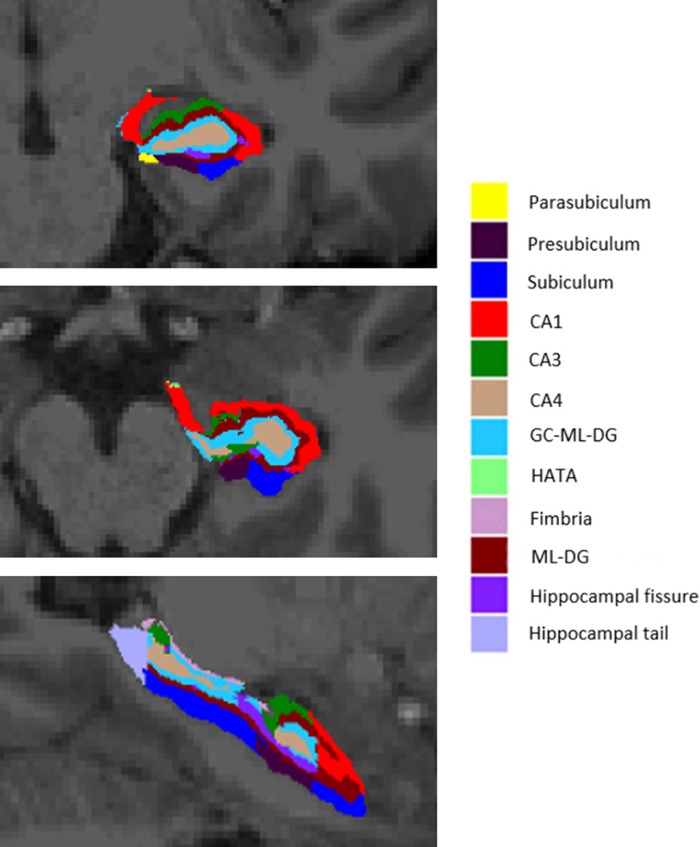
Cross-sectional slices of a T1-weighted image from one participant with automated segmentation of hippocampal subfields overlaid. Coronal (top), axial (middle), and sagittal (bottom) views. CA, cornu ammonis; GC-ML-DG, granule cells in the molecular layer of the dentate gyrus; HATA, hippocampal-amygdala transition area; ML-DG, molecular layer of the dentate gyrus.
